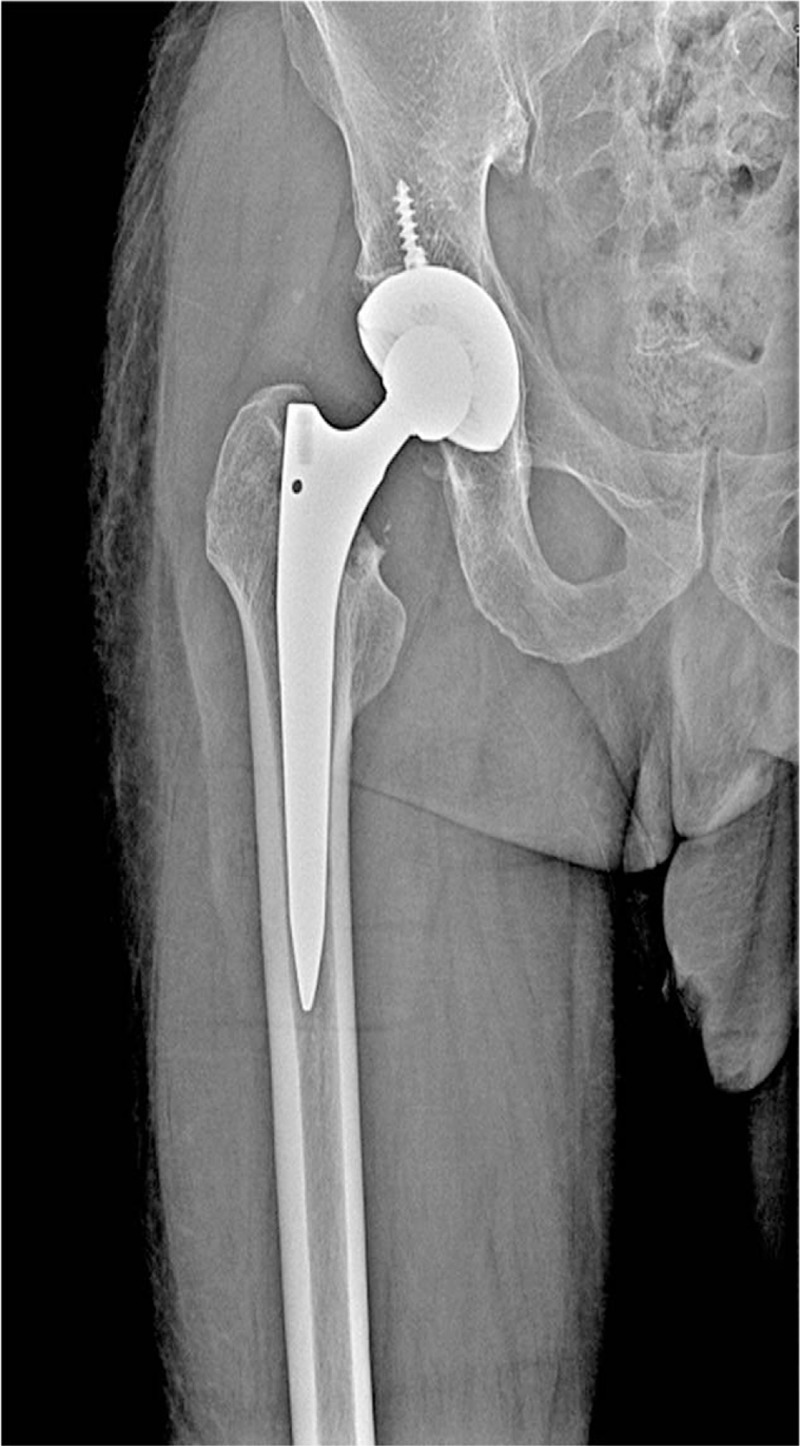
X-ray image after the third THA on the right side. THA = total hip arthroplasty.
